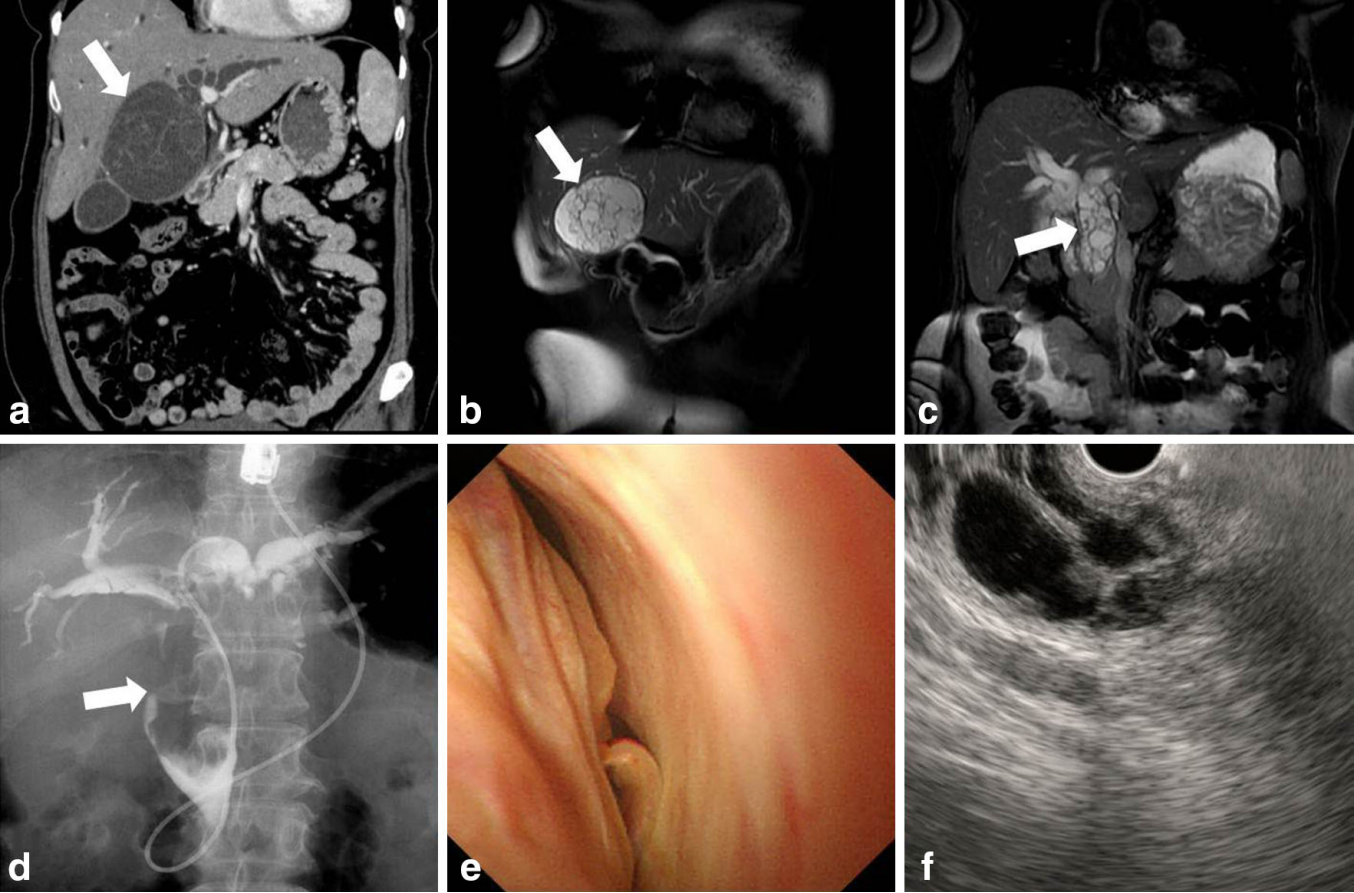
a Contrast abdominal CT revealed a multi-locular cystic lesion in the left hepatic lobe (S4), with multiple septal formations (*arrow*). Intrahepatic bile ducts were dilated. b, c MRI showed high signal intensities on T2-weighted images of the components inside the cyst (*arrow*). The septal formation was connected to the left hepatic duct and common bile duct (*arrow*), and thus the spread of the tumor into the bile duct was suspected. d ERCP revealed an oval-shaped filling defect that appeared to fill the common bile duct (*arrow*). e Cholangioscopy enabled a direct observation of the tumor filling the common bile duct. f EUS revealed that the tumor occupied the lumen of the common bile duct, and many septal formations were observed
